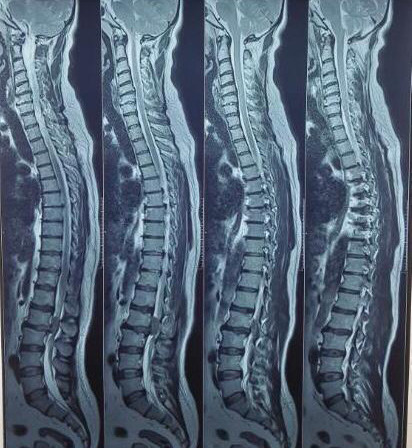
T2 sagittal section MRI of the cervical and thoracic spine.
